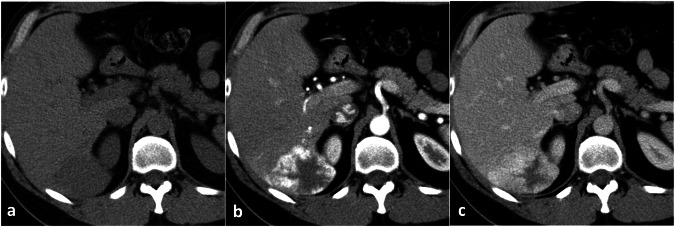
Typical CT findings in cavernous hemangioma. On unenhanced CT (a) the lesion appears slightly hypodense in comparison to liver parenchyma. After contrast material administration (b, c), an incomplete globular centripetal progressive enhancement pattern should be observed
